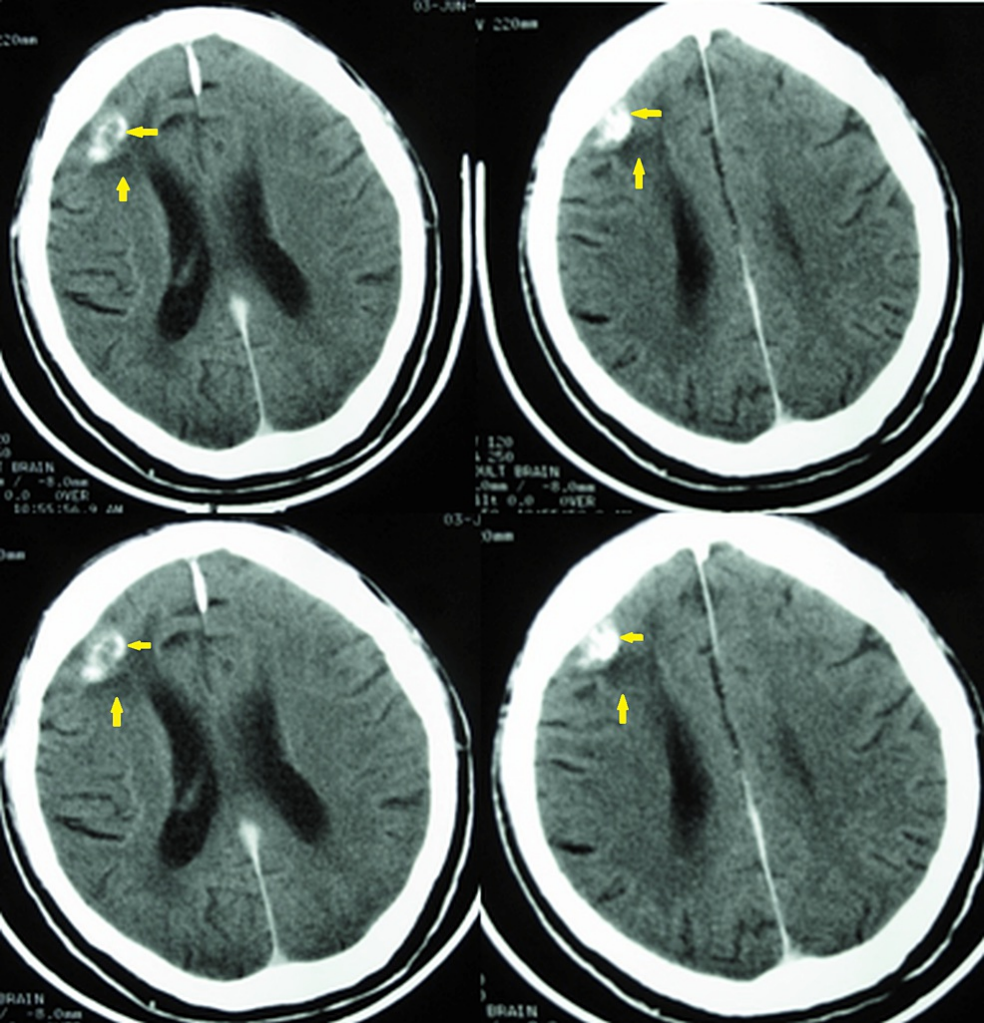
Brain computed tomography scan on October 7, 2009. Axial post-contrast images revealing a further reduction in the size of the right frontal lobe lesion to 1.9 cm × 1 cm. Images display focal calcification at the site of previous metastasis with no enhancement or perifocal edema (horizontal arrows). Low-density area behind the calcification with little expansion of the ipsilateral lateral ventricle body due to gliosis (vertical arrows).
